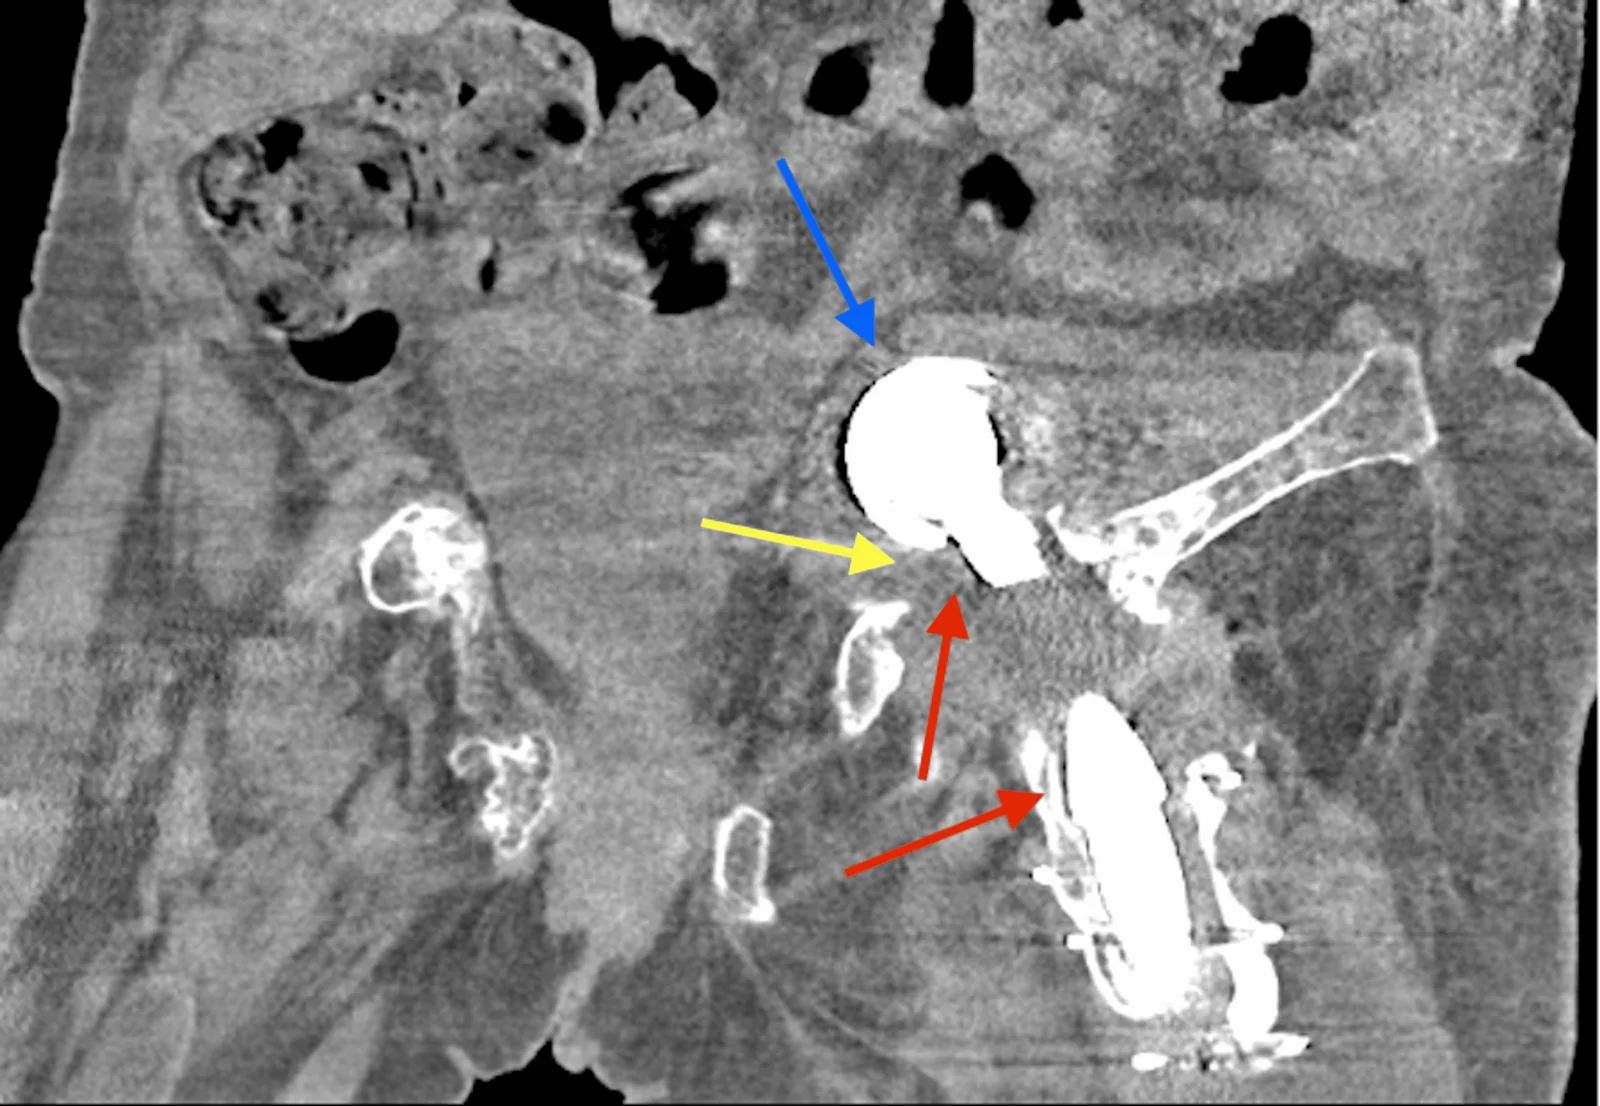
Coronal CT on soft tissue window. The image reveals an acetabular defect (yellow arrow), osteolysis (red arrows), and intrapelvic migration of the prosthetic femoral head and neck (blue arrow).
